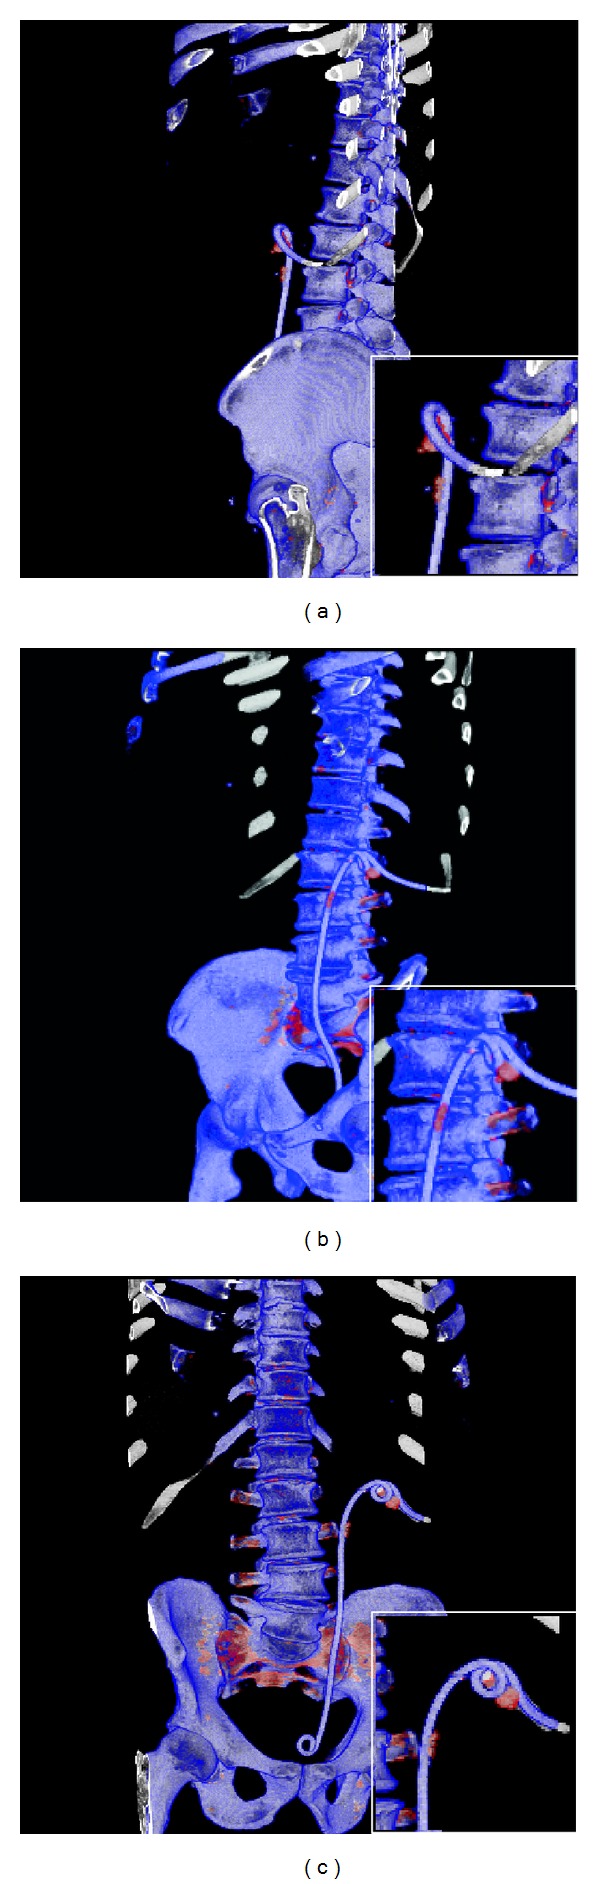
Three-dimensional dual-energy computed tomography (3D DECT) images, viewed from different angles ((a), (b), and (c)) to identify the stone/stent boundary and assess the stone's shape and volume.
